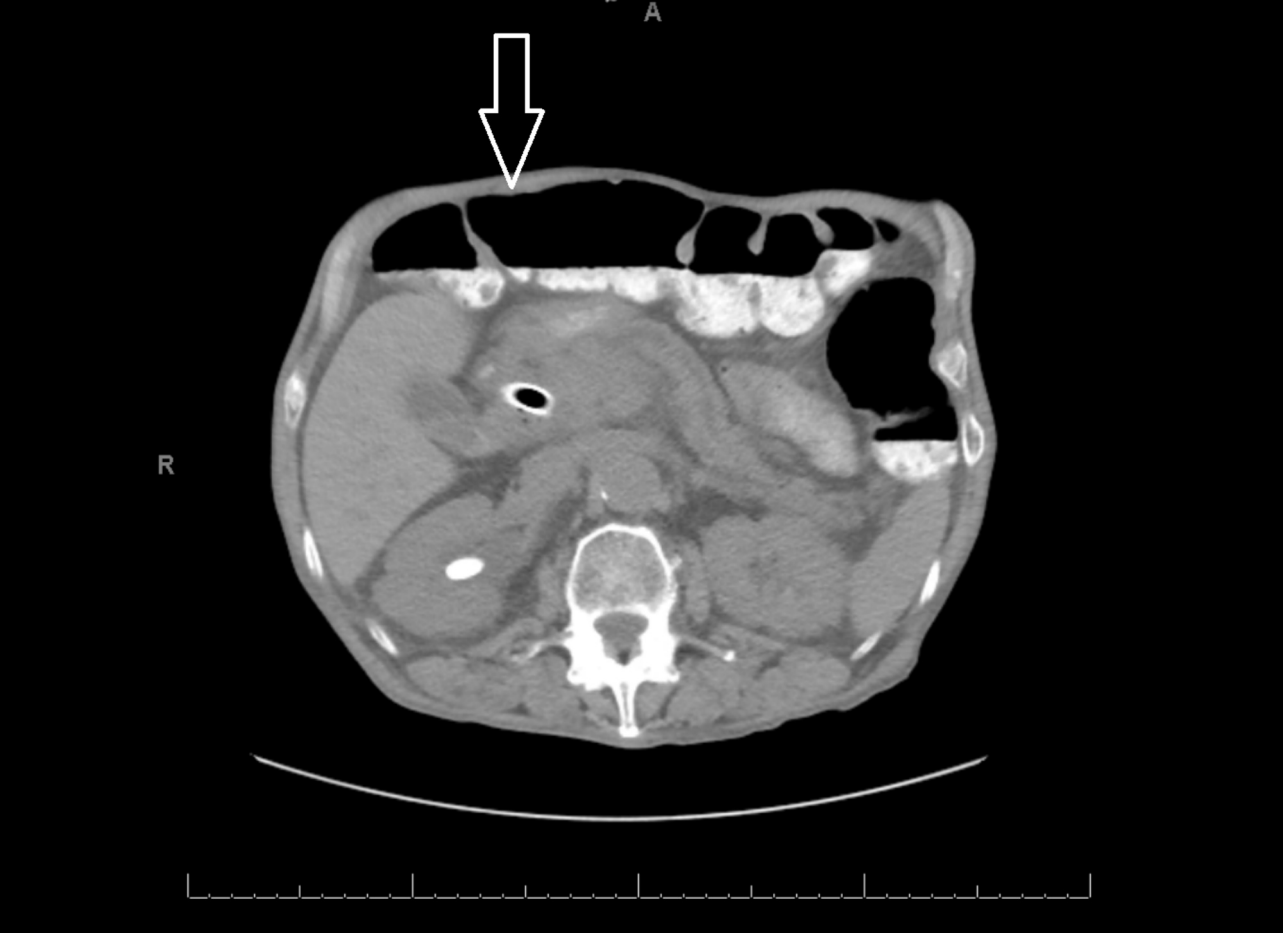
Axial CT scan two months after discharge showing resolution of the pneumoperitoneum (solid arrow) CT: computed tomography
